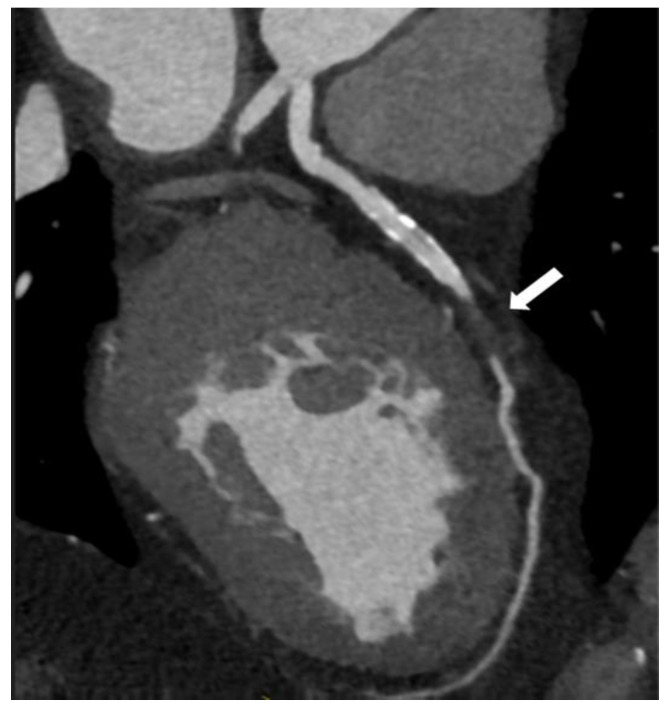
Coronary computed tomographic angiography (CCTA), oblique view, and maximum intensity projection (MIP) present the left anterior descending artery with a total occlusion in the mid-segment of the vessel (white arrow). Copyright: the authors.
